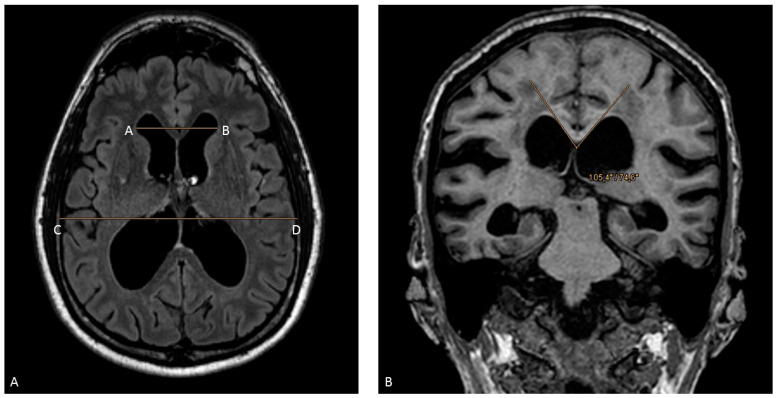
Manual measurements of the Evans’ index: (A) The ratio between the maximum width of the frontal horns of the lateral ventricles (line from A to B) and the greatest internal diameter of the skull (line from C to D) on the same axial plane, in an MRI image. The corpus callosal angle (B) measured on a coronal image perpendicular to the anterior commissure–posterior commissure (AC-PC) plane at the level of the posterior commissure.
